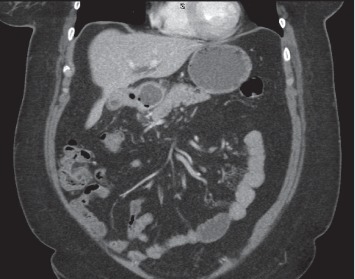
Addition CT scan showing a large stone in the duodenum and a cholecystoduodenal fistula.
